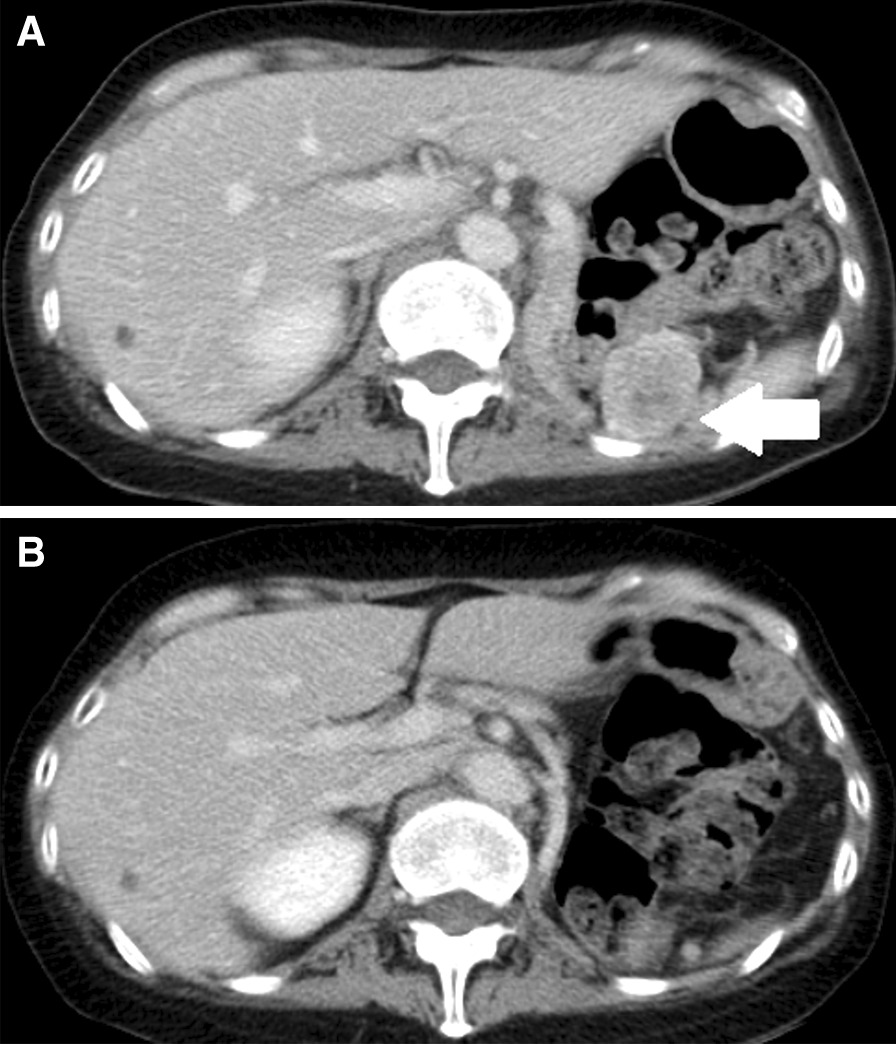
Change of CT findings of the recurrence in the left renal bed over time. A Pretreatment, 33 × 31 × 34 mm^3^ in size (white arrow). B After 12 months of immune checkpoint inhibitor therapy, the lesion size had reduced to 12 × 15 × 13 mm^3^
